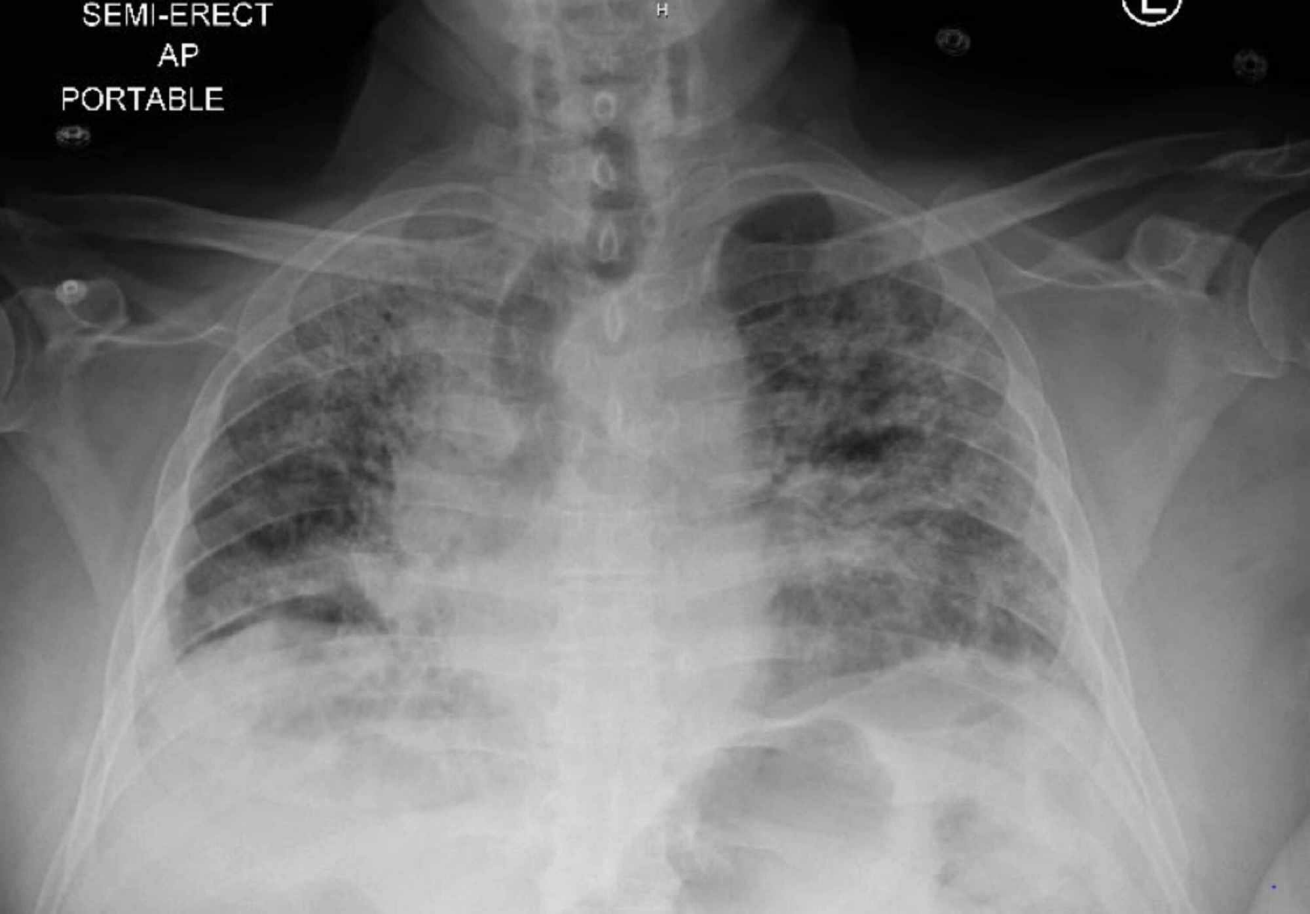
Chest X-ray showing bilateral interstitial infiltrates
